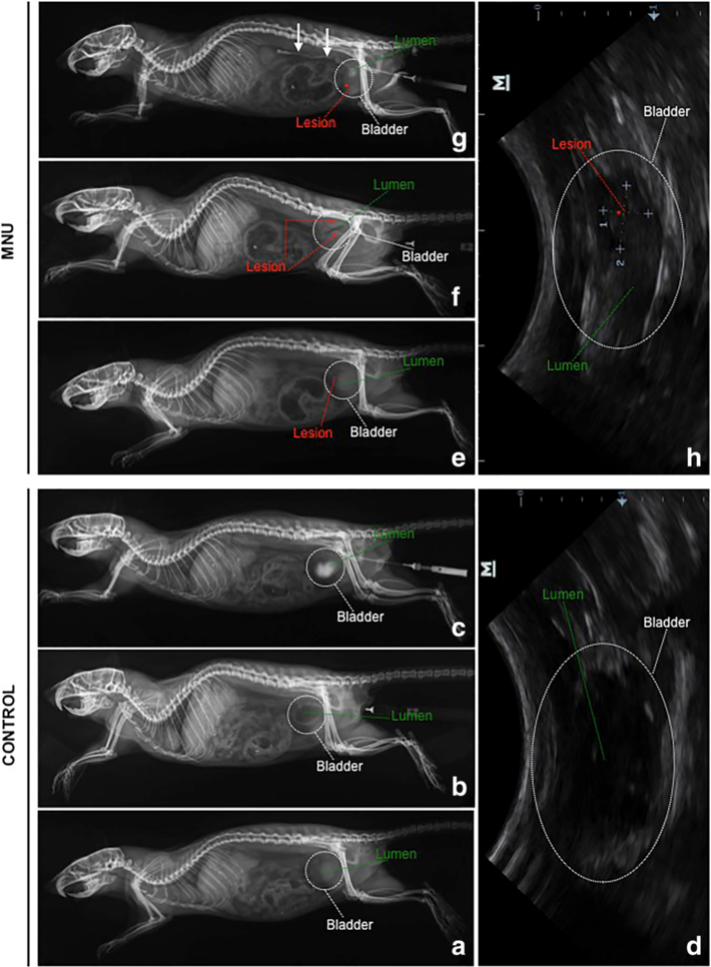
a–h Retrograde cystography and ultrasonography from CONTROL (a, b, c, d) and MNU (e, f, g, h) groups. Cystography without contrast (a), negative (b) and positive (c) contrast cystographies, and ultrasounds (d) showed no mass infiltrating the bladder walls, as well as, there were no vesicoureteral reflux and neither bladder filling defect. Cystography without contrast (e) and negative contrast cystography (f) showed a mass infiltrating the ventral, dorsal and cranial bladder walls (asterisks). Positive contrast cystography (g) demonstrated several bladder filling defects and vesicoureteral reflux unilateral (arrows). Ultrasound showed tumor (asterisk) infiltrating the bladder walls, tumor size: 1–3,9 mm, 2–5,5 mm
